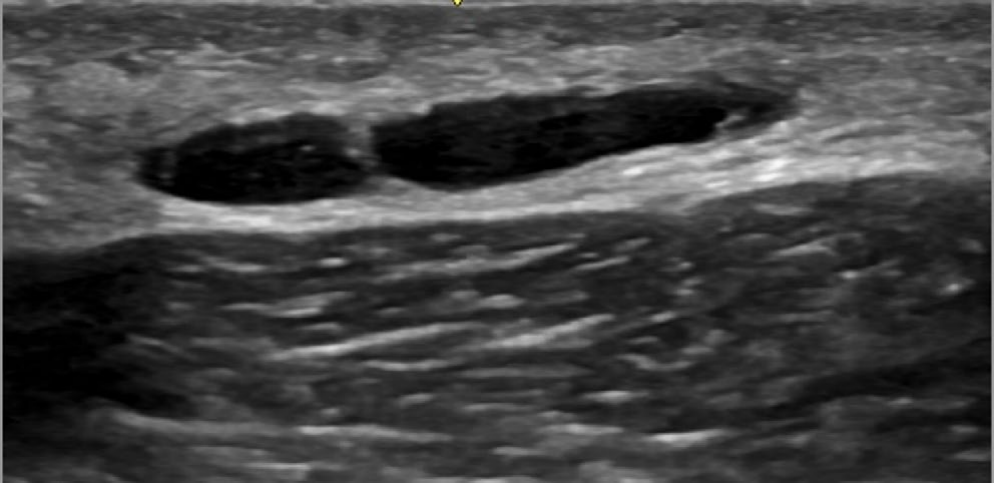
Hyaluronic acid. Ultrasound image showing an elongated anechoic deposit with thin walls in the subcutaneous layer of the gluteal region, adjacent to the muscular fascia. Characteristic of hyaluronic acid (HA) filler.
